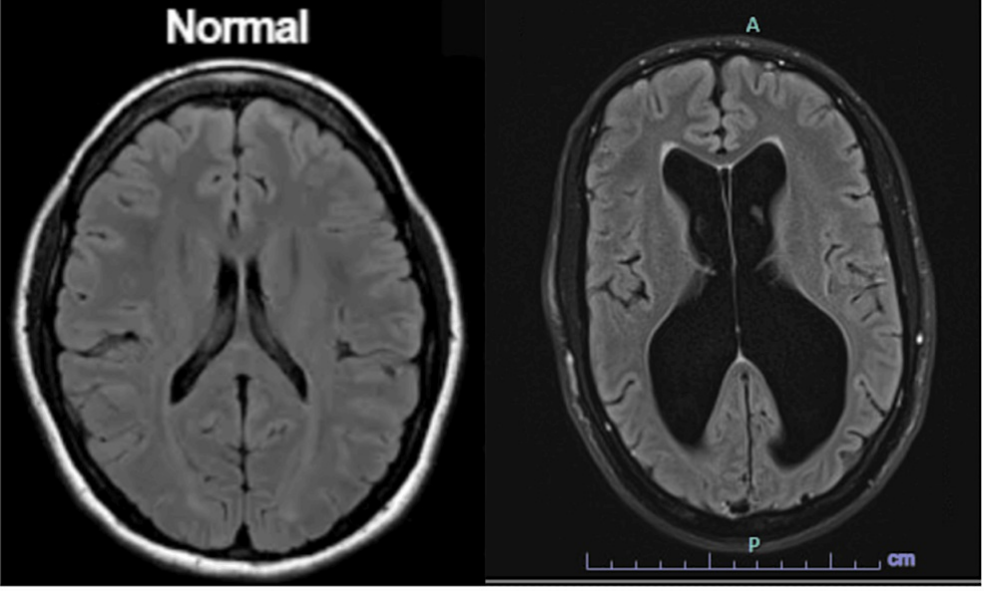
Normal ventricular size versus the MRI of our patient's ventricles exhibiting severe ventricular dilation causing normal pressure hydrocephalus MRI: magnetic resonance imaging
